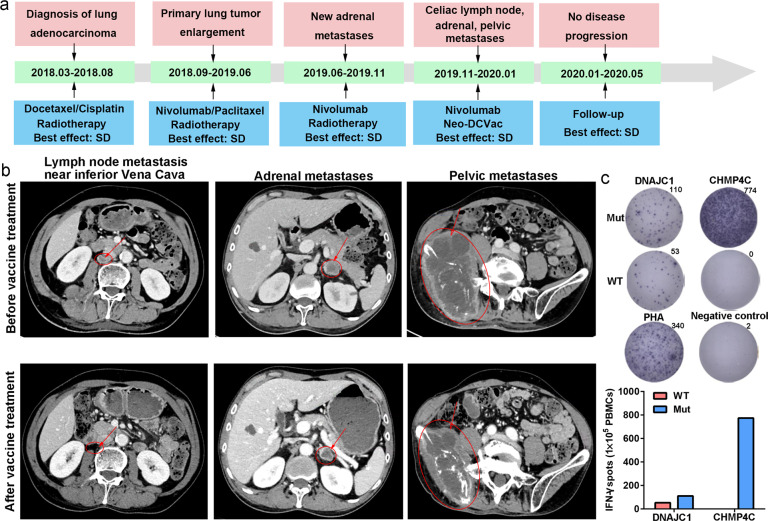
Clinical and immune responses to personalized Neo-DCVac in patient 17. a Clinical course of the disease and previous treatments of patient 17. The patient failed three lines of chemotherapy, radiotherapy and PD-1 inhibitor, and his tumor target lesions were reduced by 29% following Neo-DCVac administration. b Computed tomography (CT) scans were performed before and after personalized Neo-DCVac immunotherapy, and representative images are shown. c Autologous PBMCs were stimulated with 15 candidate mutant peptides for 10 days, after which IFN-γ ELISpot assays were performed to assess the T-cell-specific antigen response
